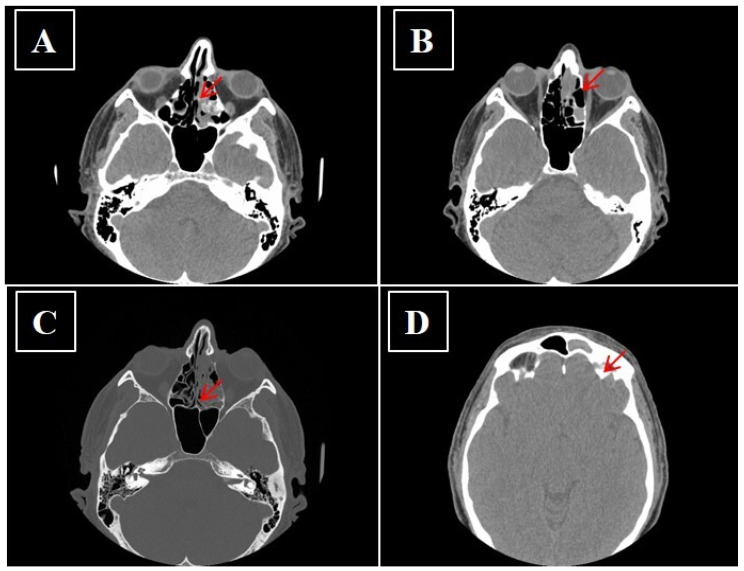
(A–D) No-contrast media CT showing sinonasal neuroendocrine tumors—MENs of ethmoidal cells and to left frontal sinus before surgery (arrow).
